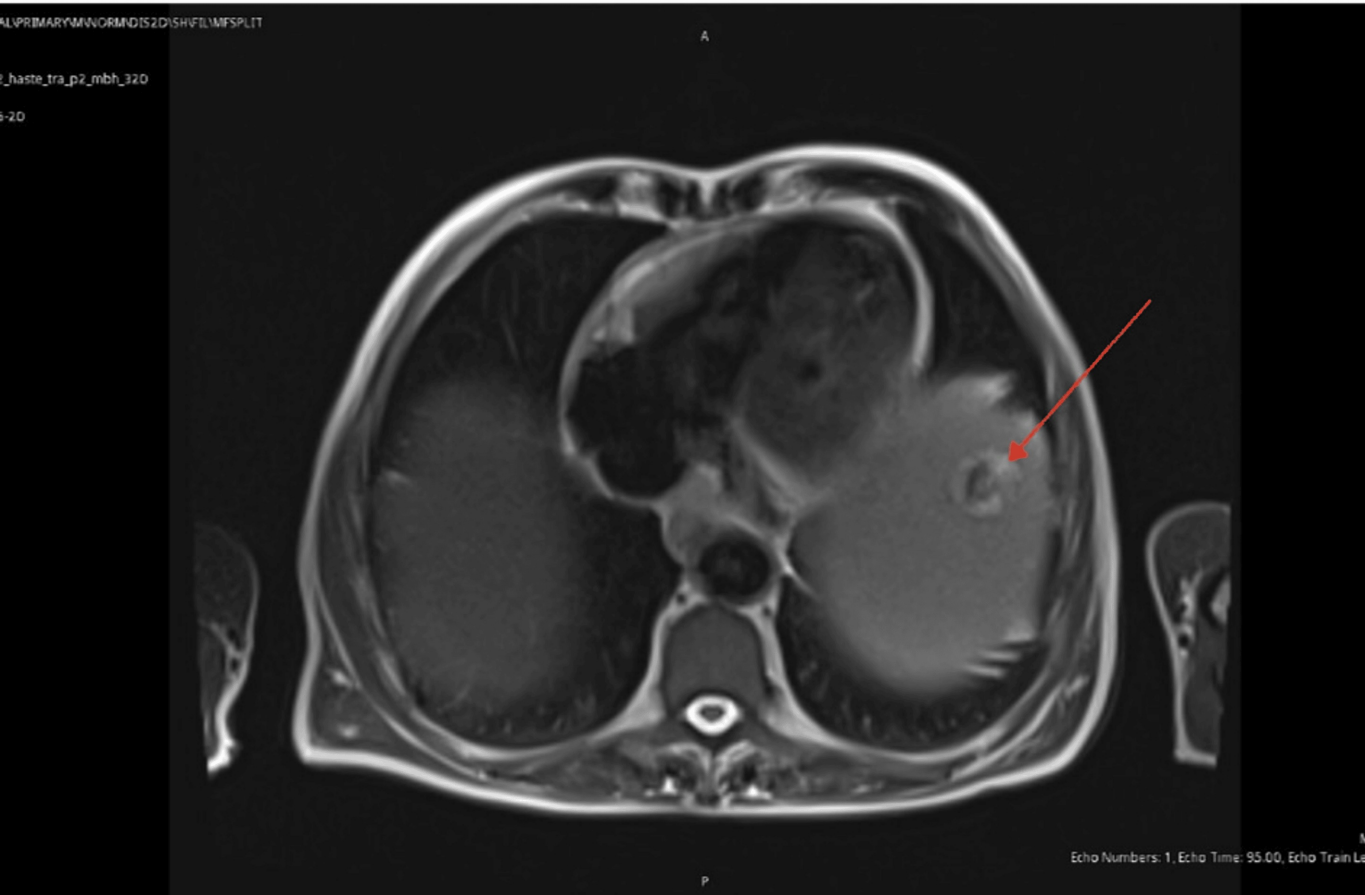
Magnetic resonance imaging (MRI) of liver and spleen showing largest of three splenic lesions (red arrow), other two lesions are not shown
